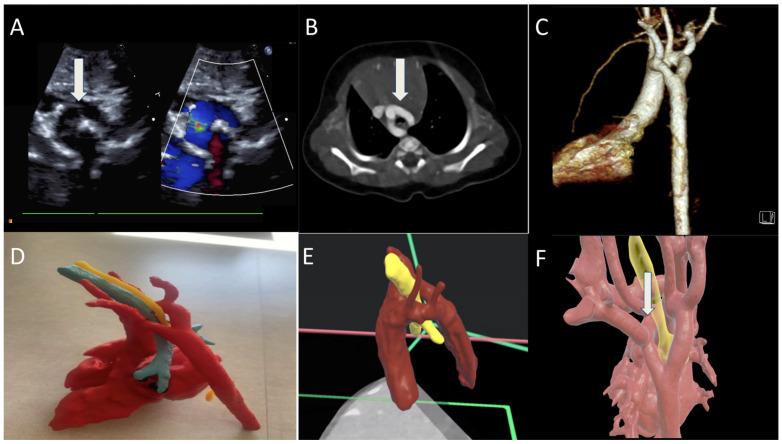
(A) TTE 2D with color showing double aortic arch around the airway; (B,C) CT of chest with double aortic arch encircling the trachea; (D) 3D-printed model of the DAA (red), teal is trachea, and yellow is the esophagus; (E,F) VR models detailing the double arch (red) and trachea (yellow) encircled by the vascular anomaly.
